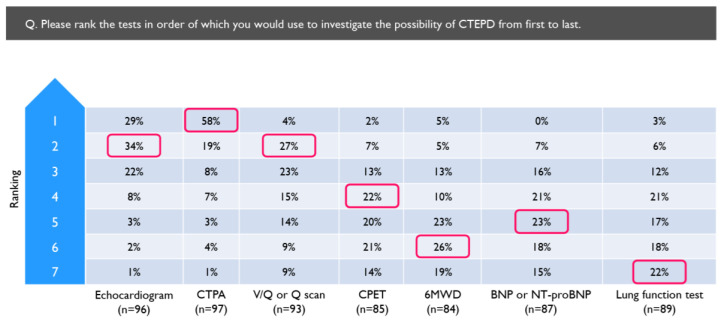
Tests ranked in the order they would be used to investigate for CTEPD by physicians who routinely investigate for CTEPD following PE (*N* = 97). Red boxes indicate the most common ranking allocated to each investigation. 6MWD = 6 min walking distance; BNP = brain natriuretic peptide; CPET = cardiopulmonary exercise test; CTPA = computed tomography pulmonary angiogram; NT-proBNP = N-terminal pro-brain natriuretic peptide; Q = perfusion; V/Q = ventilation/perfusion.
